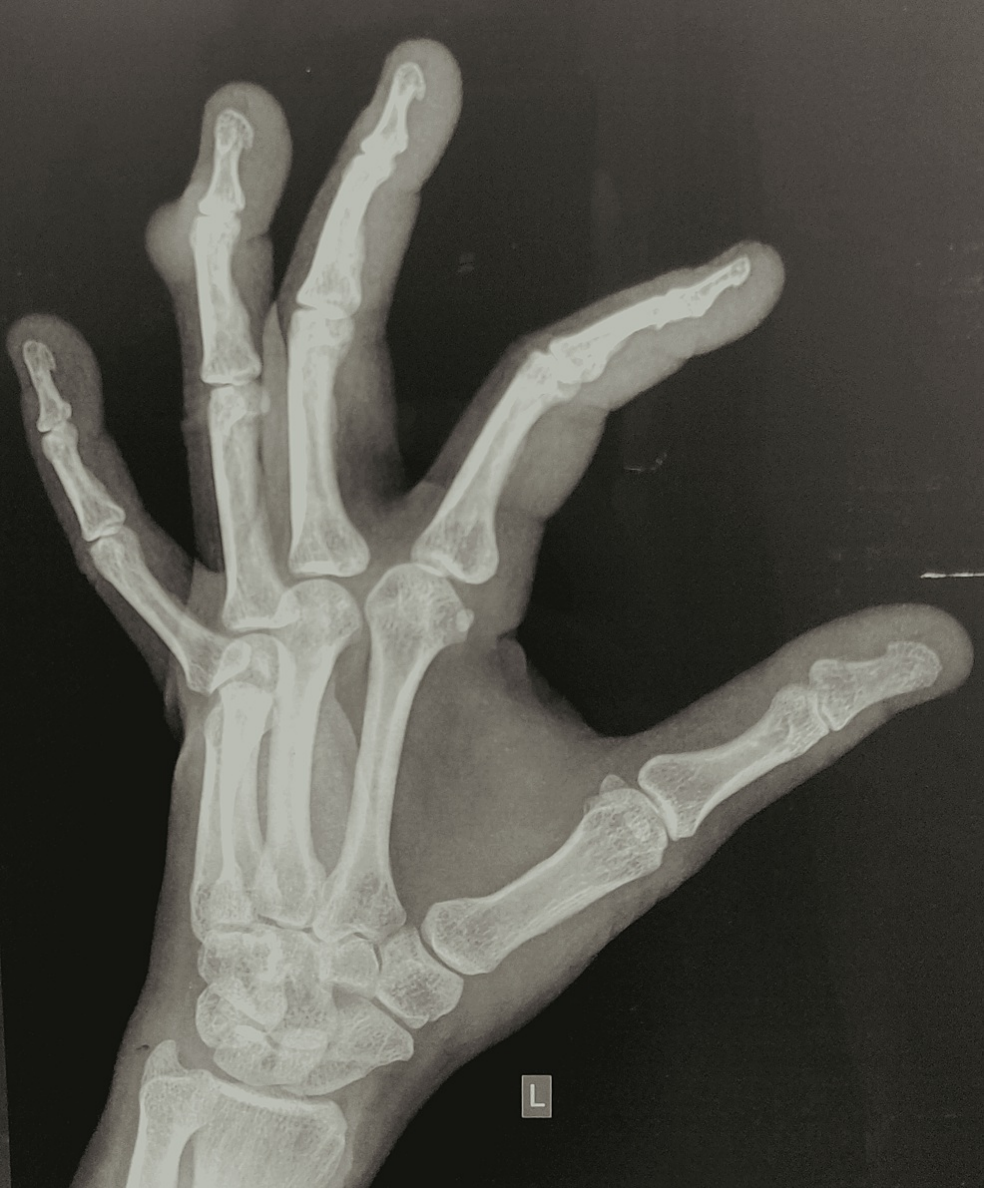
X-ray of left hand showing soft tissue shadow in ring finger
